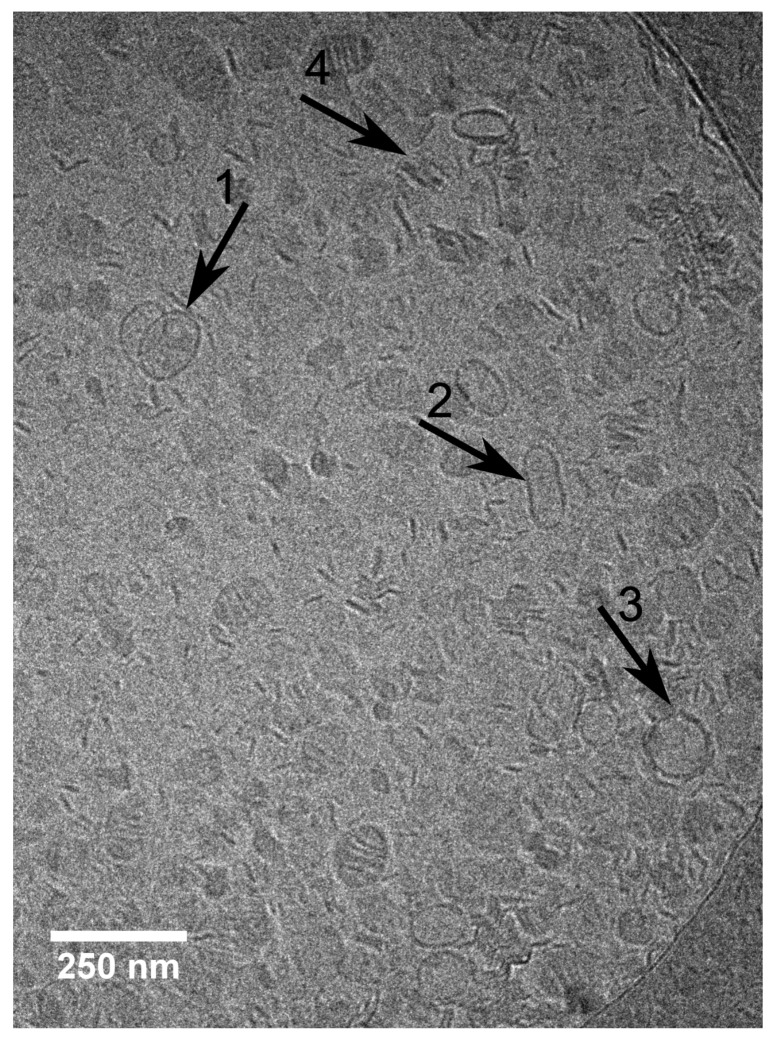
Cryo-TEM image of a sample with 4 mol% β-aescin prepared at 10 ${}^{\circ }$C. The arrows point on deformed SUVs (1), elongated SUVs (2), SUVs with edges (3) and stacks of bilayer disks (4). Reproduced from Ref. [48] with permission of Elsevier.
